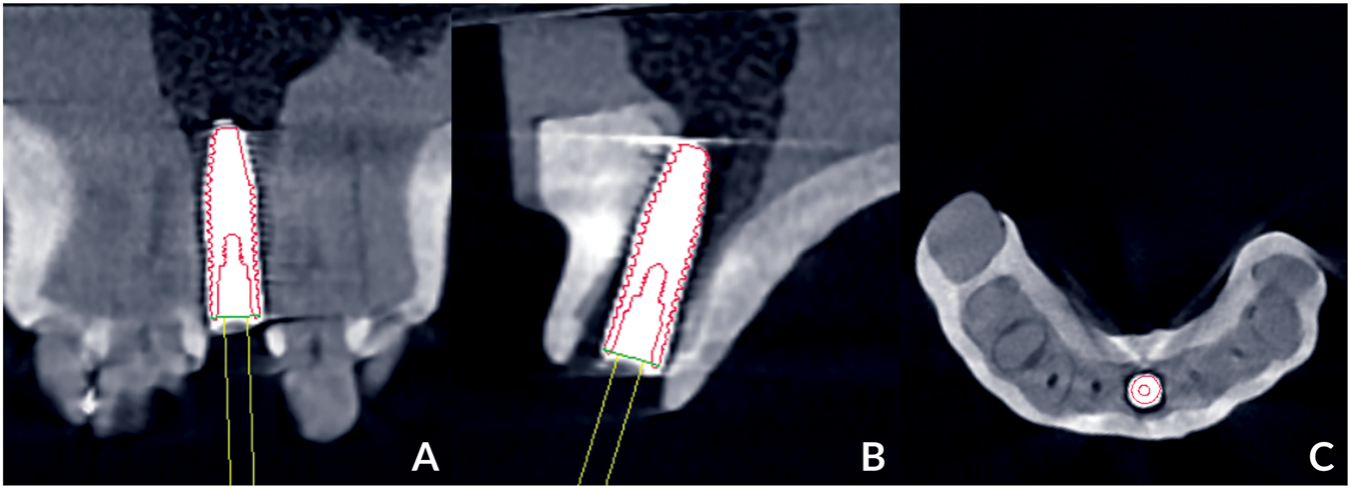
Locating the actual implant position in the “Treatment evaluation” module with CBCT slides in 3 planes. A Tangential plane, B Cross-sectional plane, C Axial plane.
